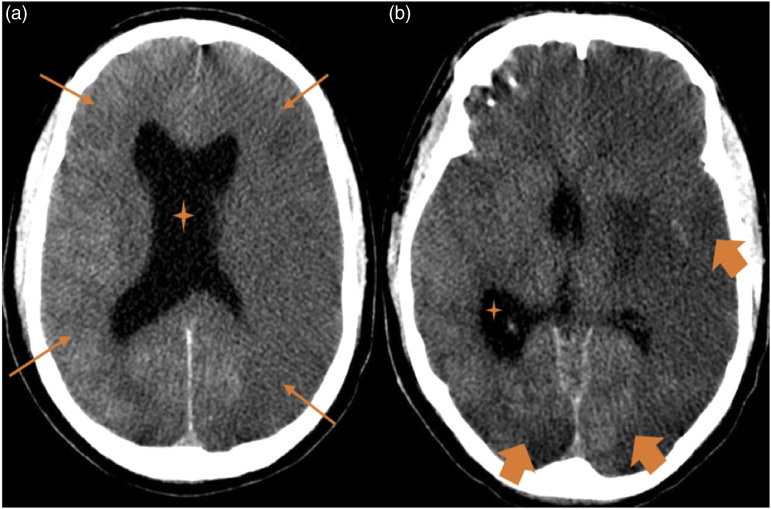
Fatal ischaemic stroke. A 23-year-old man patient with cystic fibrosis underwent double-LT and suffered two cardiac arrests during the intervention. After the transplantation, he presented non-reactive mydriasis and absence of brainstem reflexes. Figures: axial CT scan (a and b), diffuse cerebral oedema (thin arrows) and supratentorial hydrocephalus (stars). Left middle-cerebral artery and bilateral posterior-cerebral artery ischaemic strokes (thick arrows).
